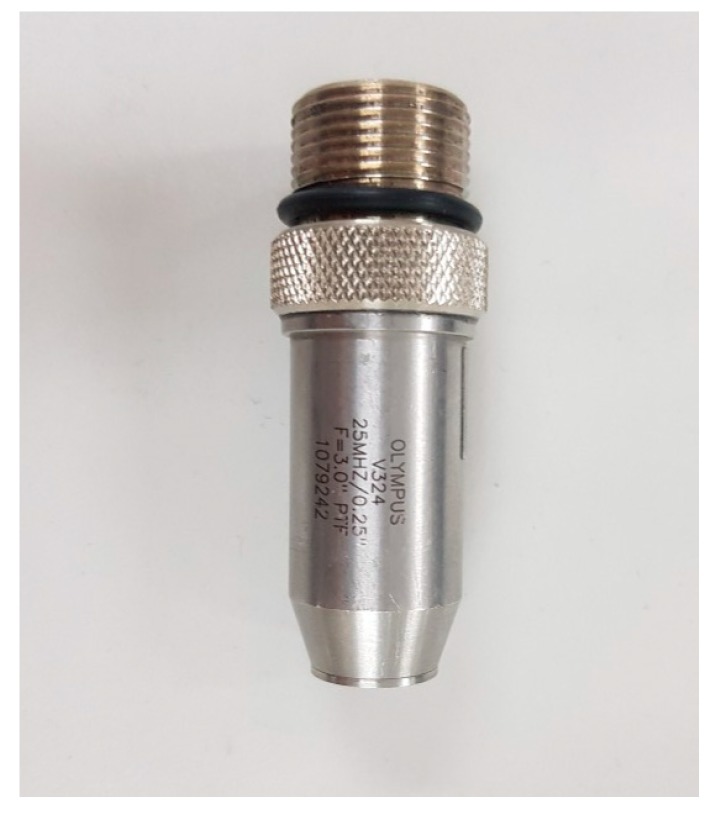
Twenty-five megahertz ultrasonic transducer.
